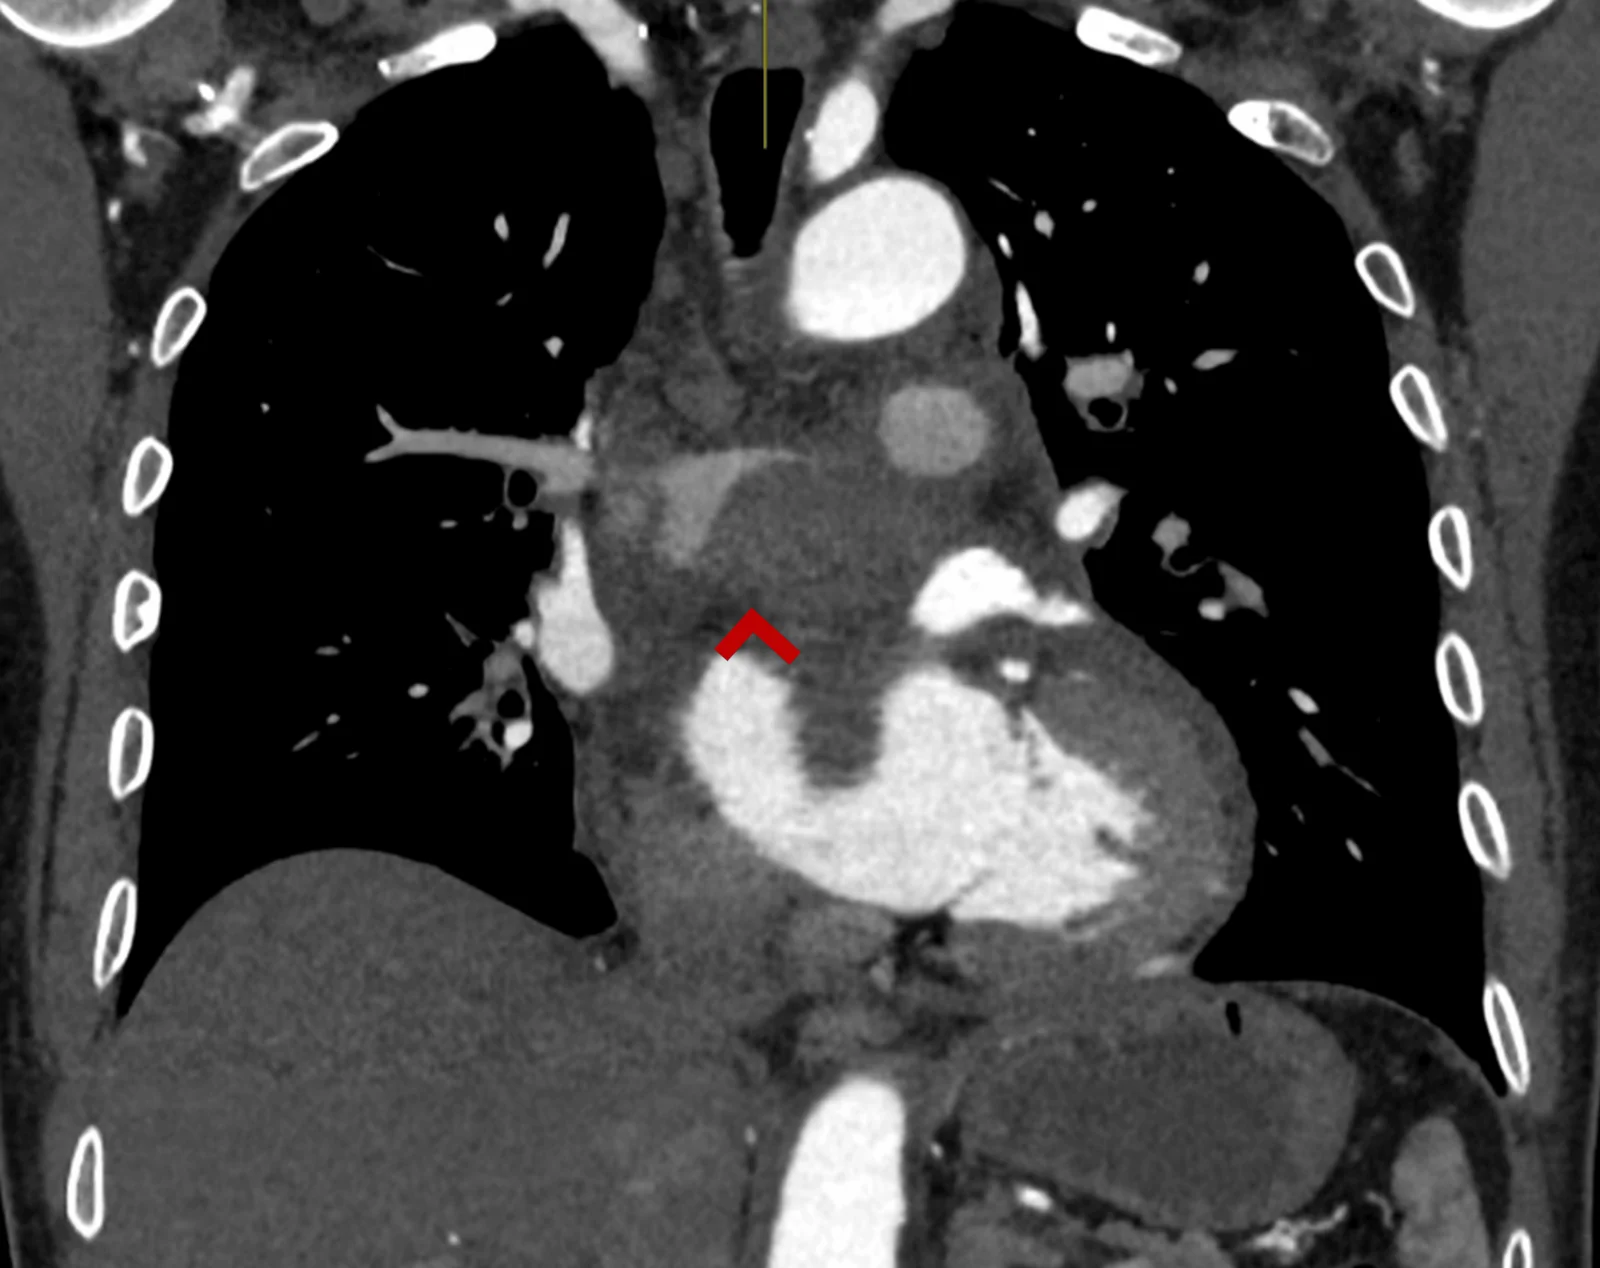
CT scan, coronal multiplanar reformat. The red arrow indicates haematoma within the sheath of the great vessels partially effacing the pulmonary trunk.
